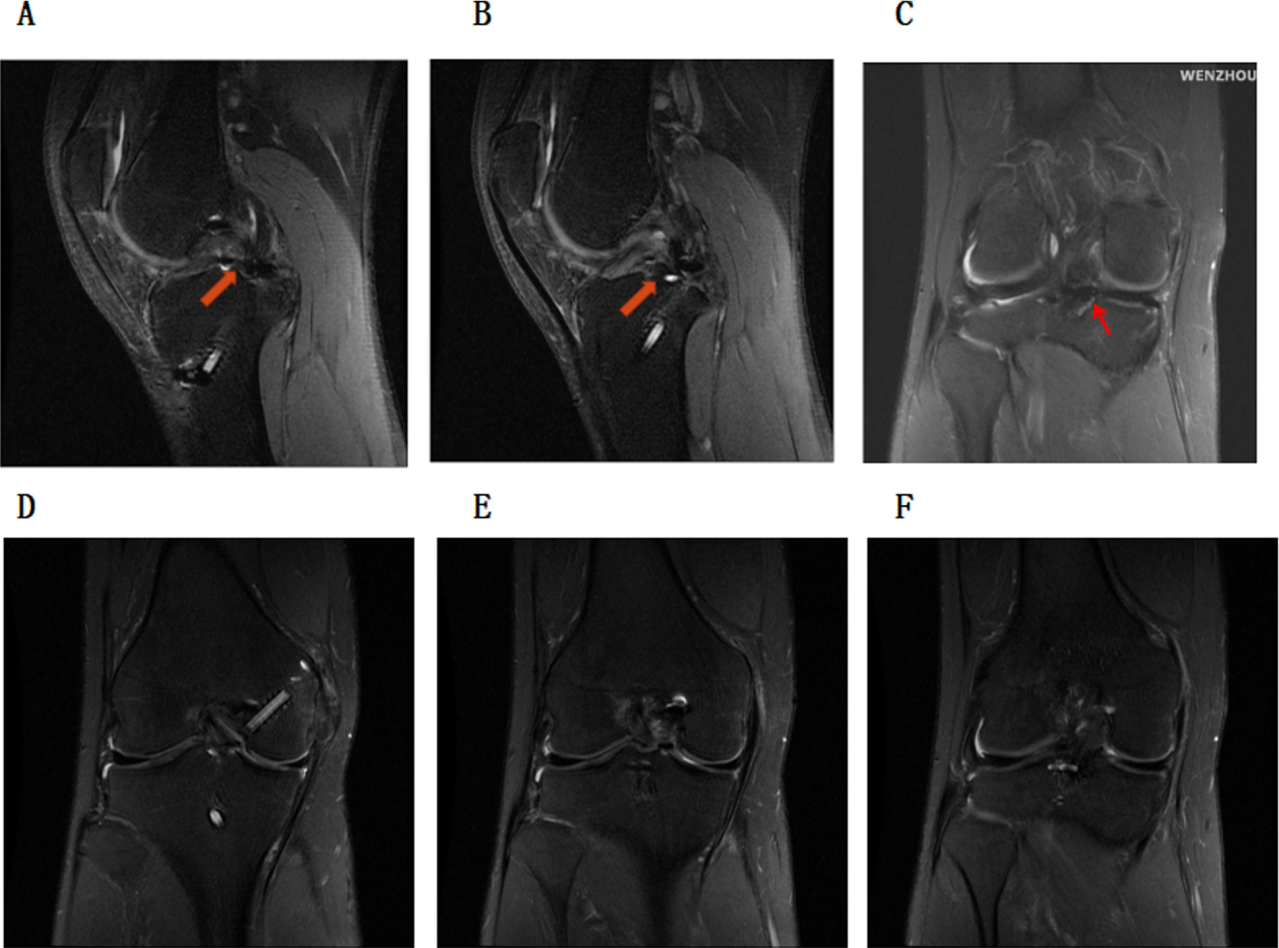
The postoperative MRI at 1 year. a–c The red arrows show the good union of the PRMM. d–f The good union of the PCL. PRMM, posterior root of medial meniscus, PCL, posterior cruciate ligament
